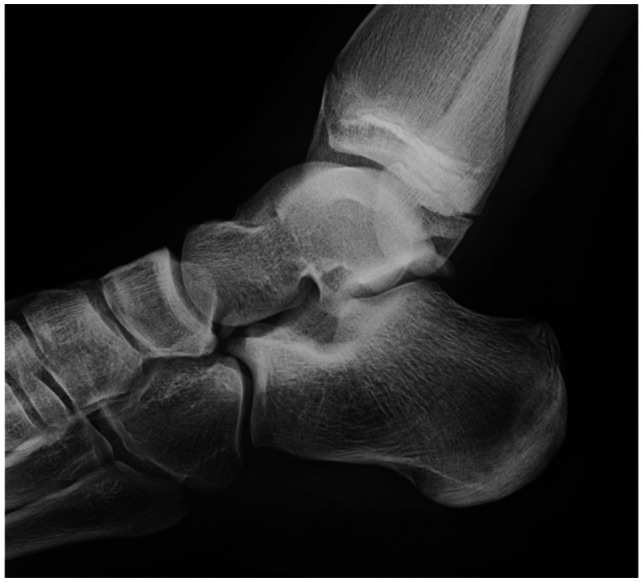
Xray 6 months after surgery confirming complete excision of the lesion without residual pathology.
